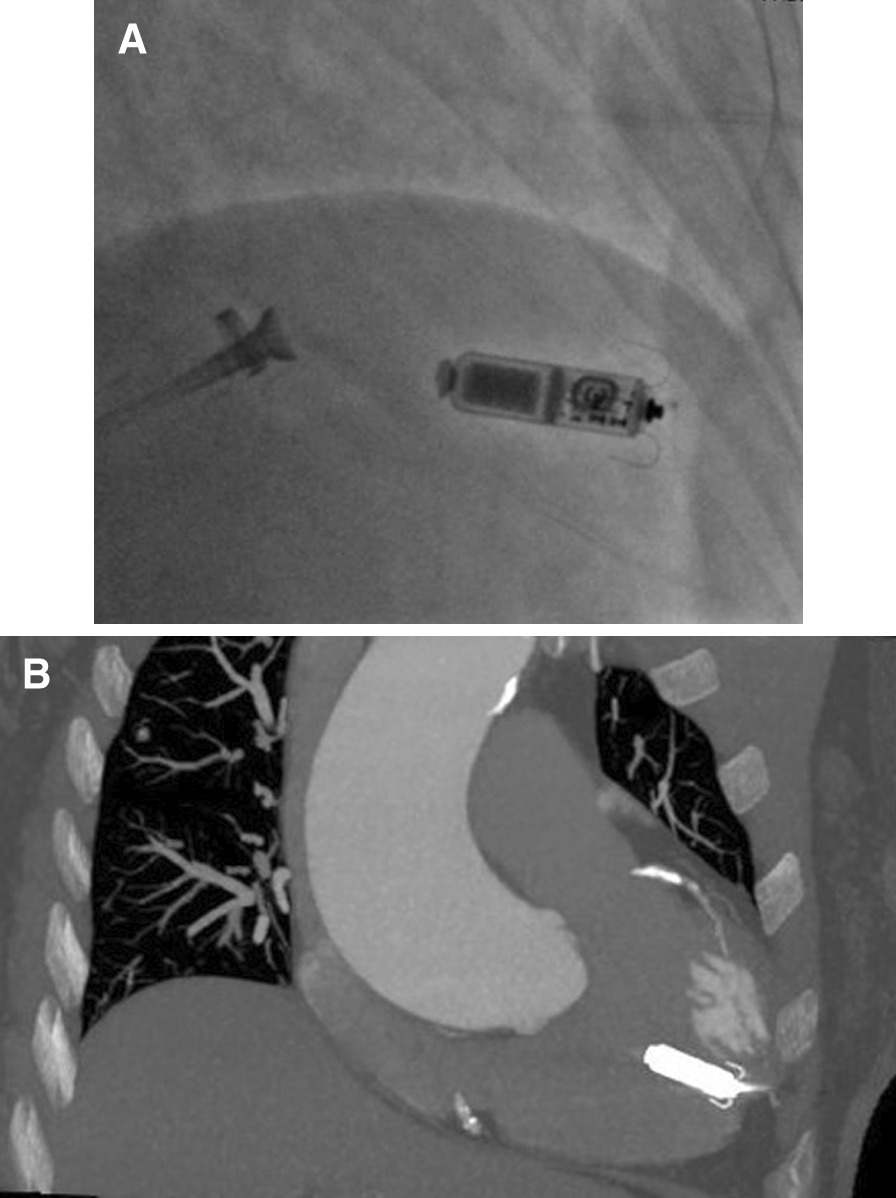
The images showing the location of Micra leadless pacemaker: A. After deployment at right anterior oblique view; B. CT scan after implantation indicating the leadless pacemaker locating at the apex of right ventricular
